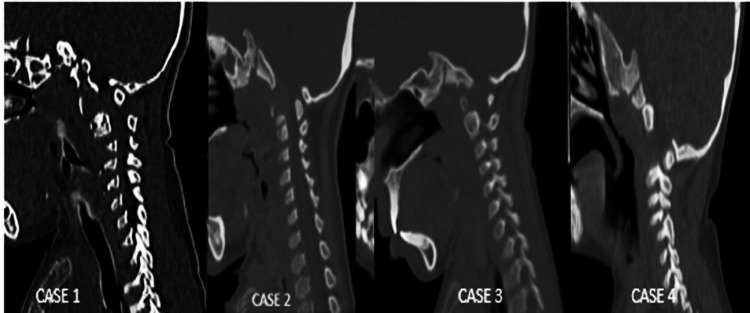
Preoperative CT of the cervical spine showing a thickened posterior arch of C1 in all patients.
